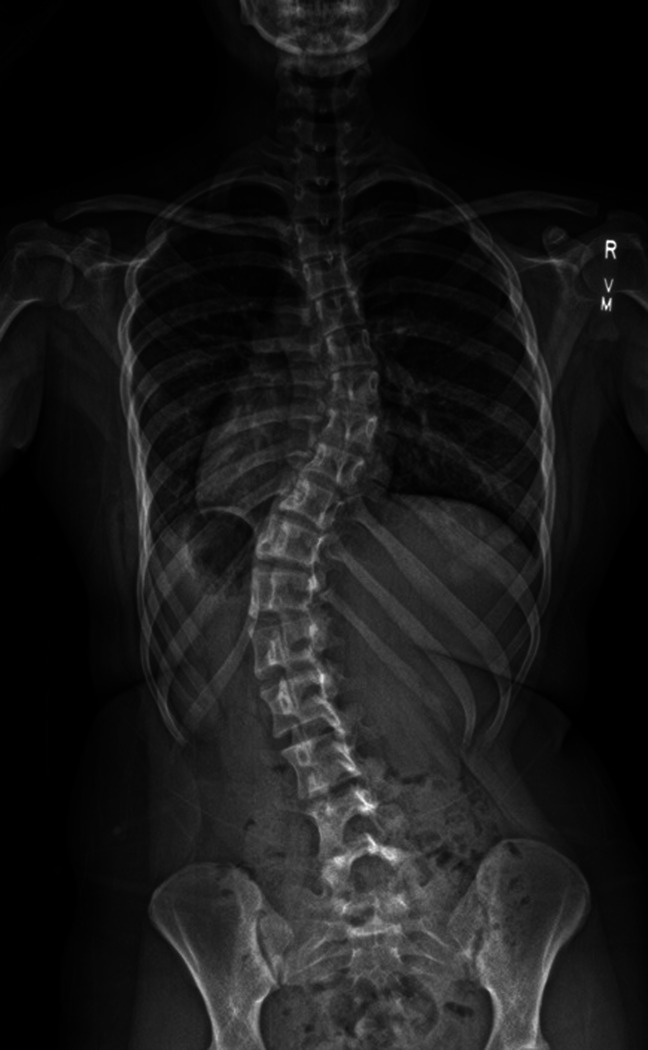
Preoperative radiograph of a patient who received erector spinae plane block with liposomal bupivacaine.
